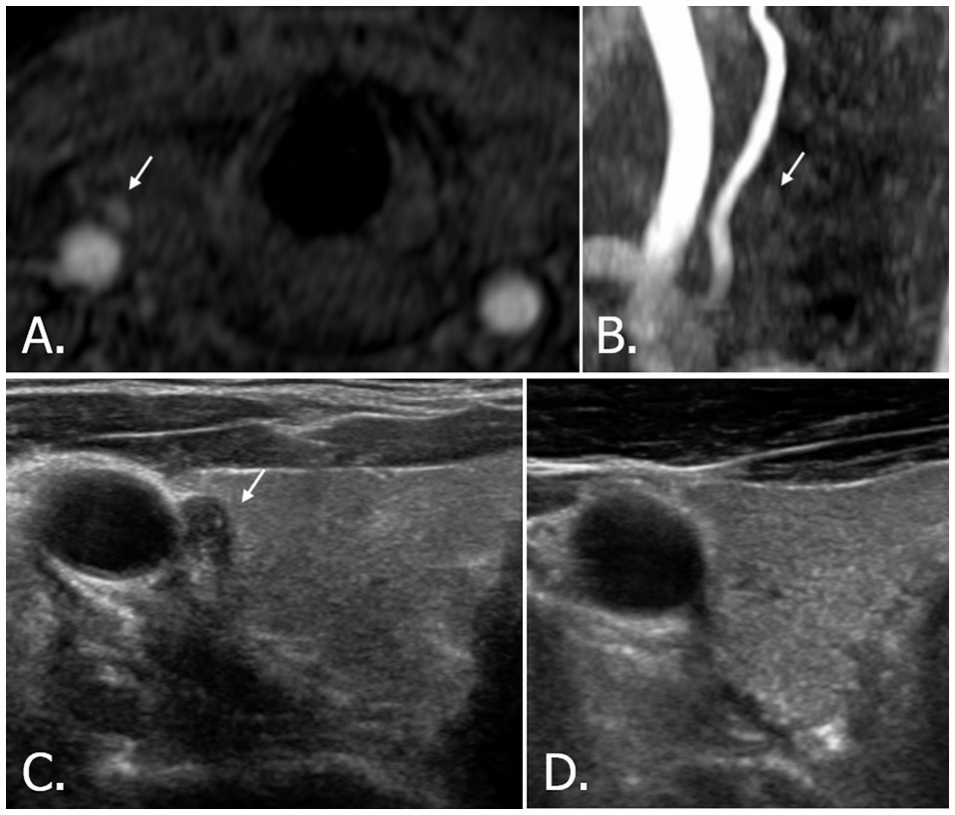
42-year-old male with right thyroid nodule. Source image (A) of TOF-MR angiography shows a small hyperintense nodule (white arrow) in the right thyroid gland. But the nodule (white arrow) is hardly detectable on MIP image (B). Transverse (C) US image of right thyroid demonstrates a hypoechoic, solid nodule (white arrow) with echogenic spots, which is classified as highly suspicious nodule (K-TIRADS, ACR-TIRADS, and EU-TIRADS category 5). A cytologic result showed insufficient cellularity, but the nodule disappeared after aspiration (D). TOF-MR, time-of-flight magnetic resonance; MIP, maximum intensity projection; US, ultrasound; K-TIRADS, Korean Thyroid Imaging Reporting and Data System; ACR-TIRADS, ACR Thyroid Imaging Reporting and Data System; EU-TIRADS, European Thyroid Imaging Reporting and Data System.
